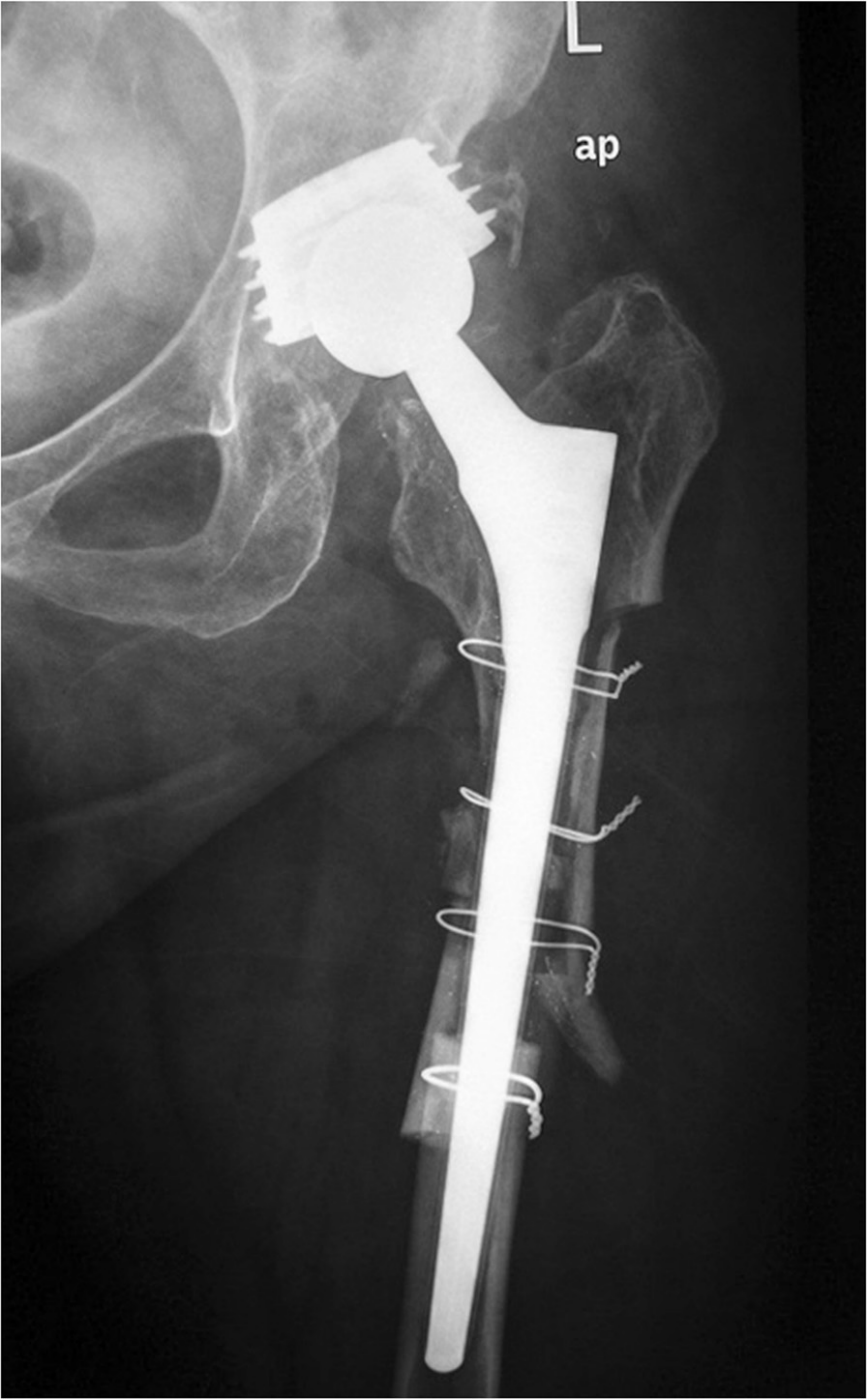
The immediate postoperative radiograph.
